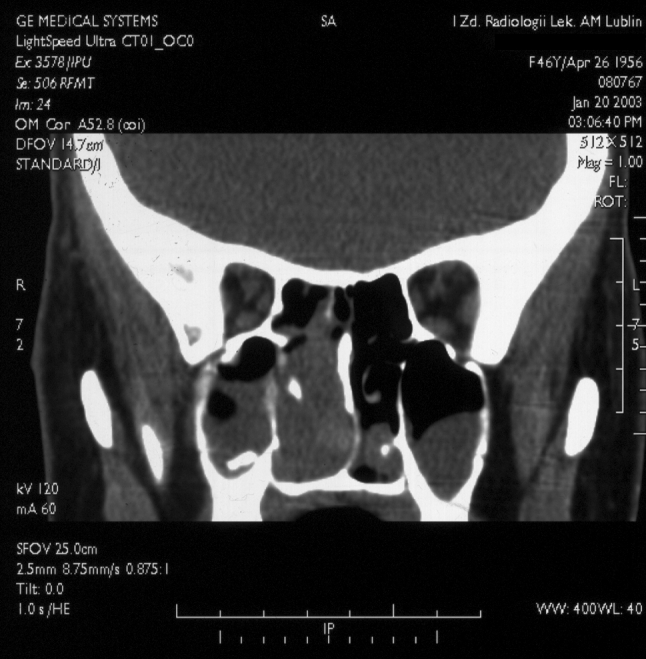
Computed tomography, coronal plane, shows homogenous tumor mass in the right nasal cavity
